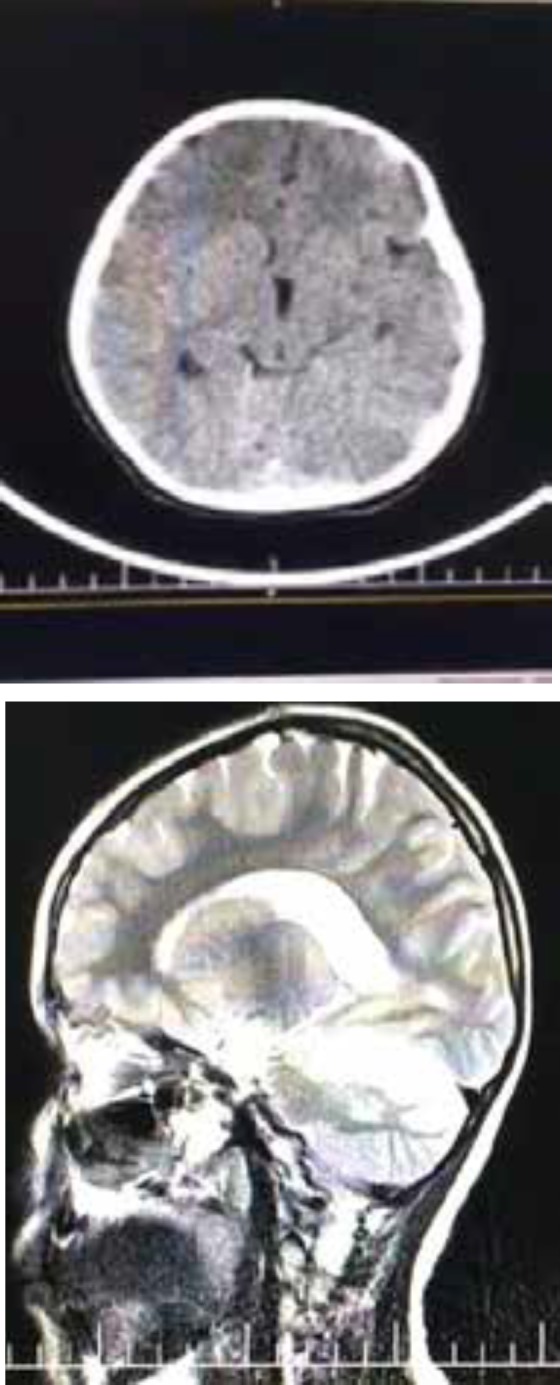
Brain CT scan without contrast (left) on first day showing dim hypodensity in the left cerebellar hemisphere and sagittal plane of brain MRI (right) on second day
